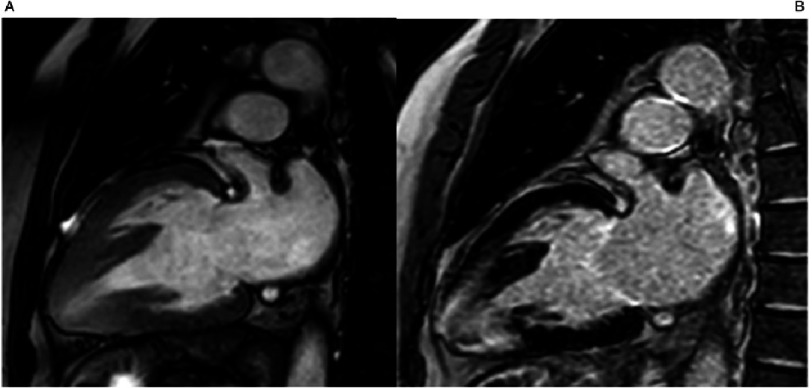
Cardiac Magnetic Resonance (CMR) Apical 2CH view: A) Cine: Apical hypertrophic cardiomyopathy with the classical “ace of spades” shape. B) Late gadolinium enhancement images: severe fibrosis in the segments of maximum hypertrophy.
